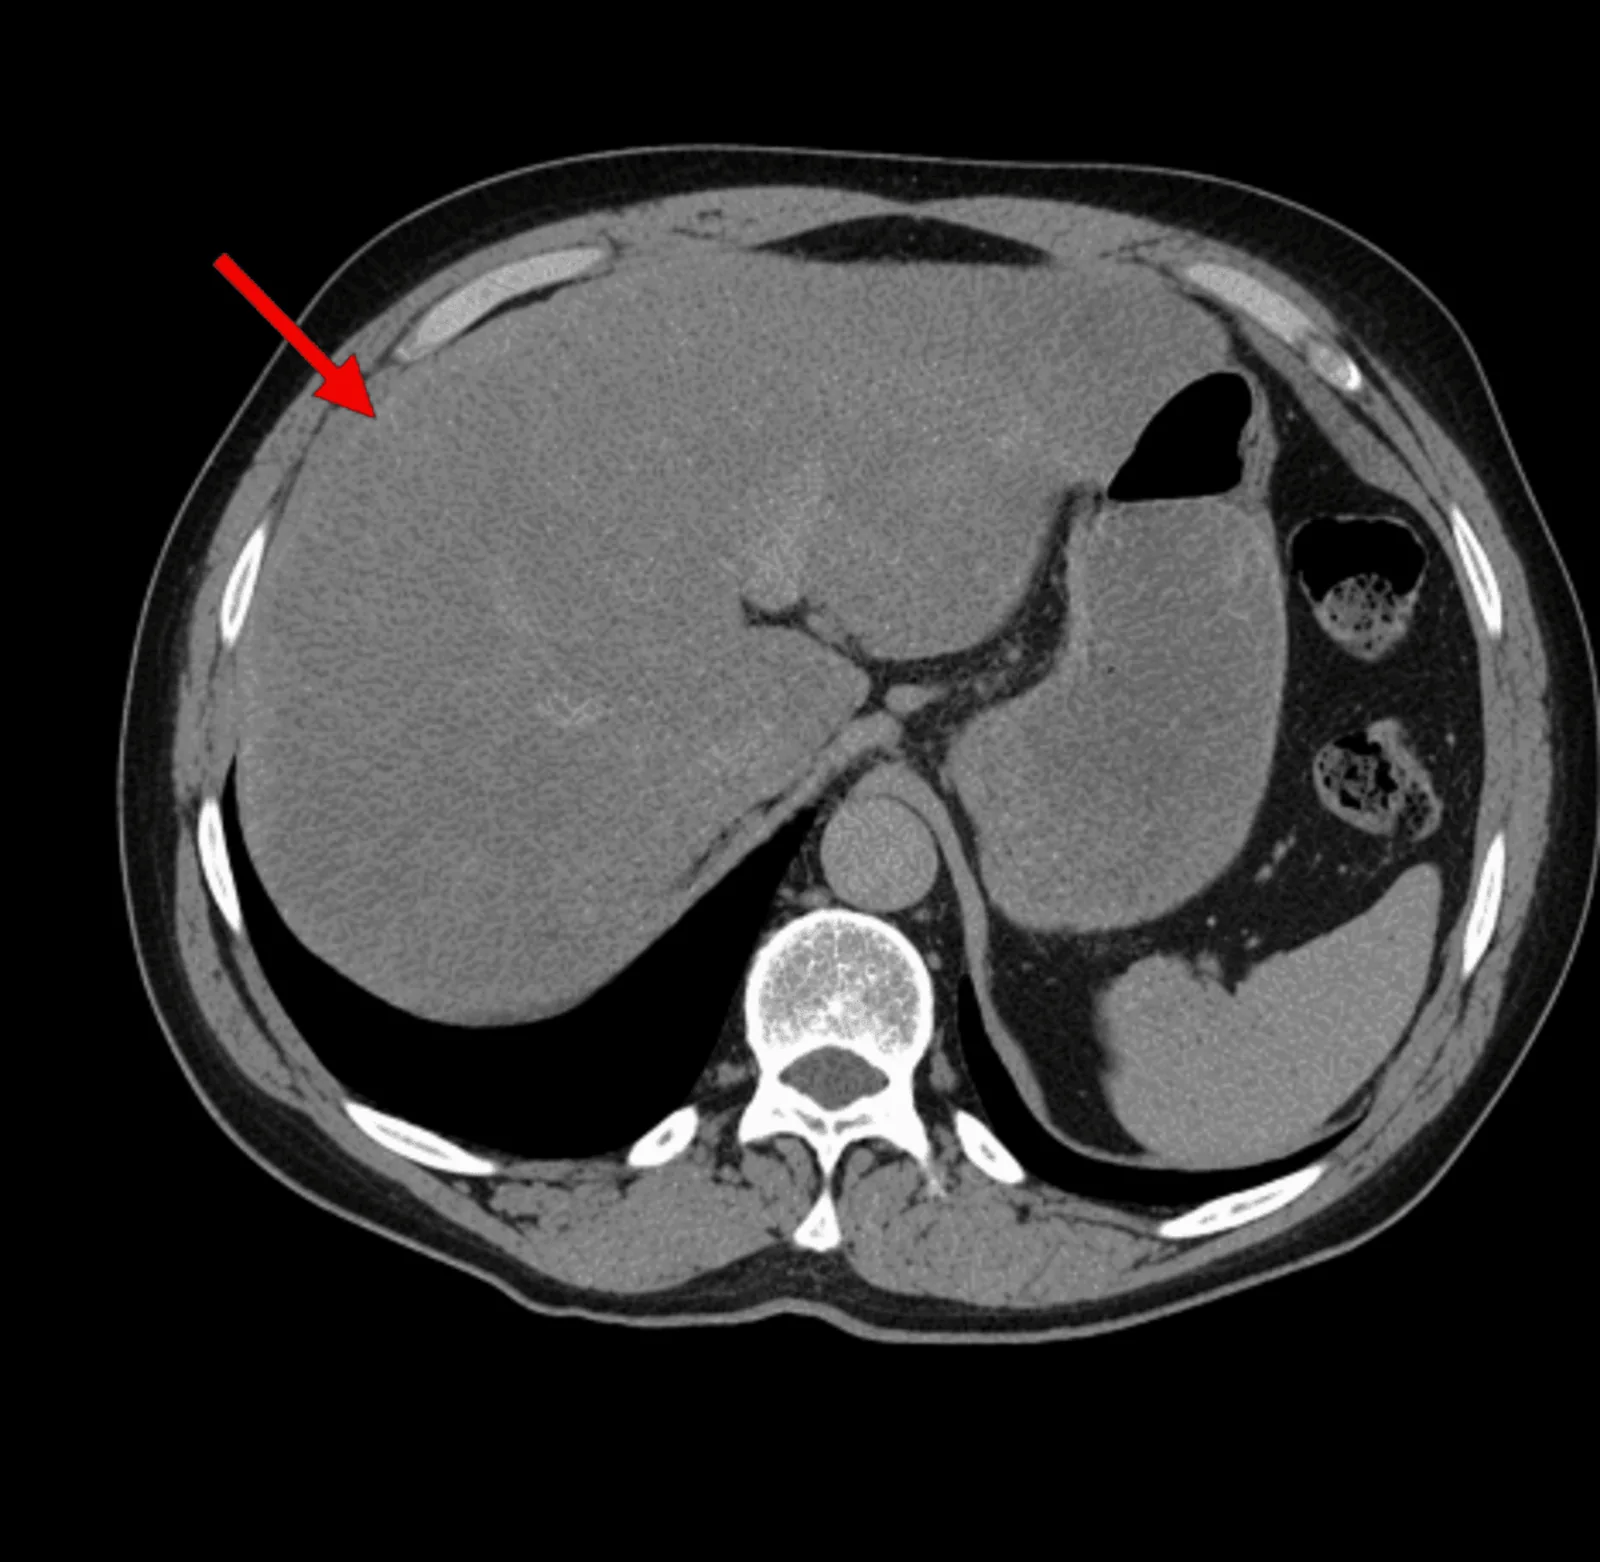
Computed tomography of abdomen and pelvis without contrast. Computed tomography (CT) of the abdomen and pelvis without contrast showing hepatomegaly with diffuse low hepatic attenuation consistent with hepatic steatosis; the liver measured 19.9 cm (red arrow).
